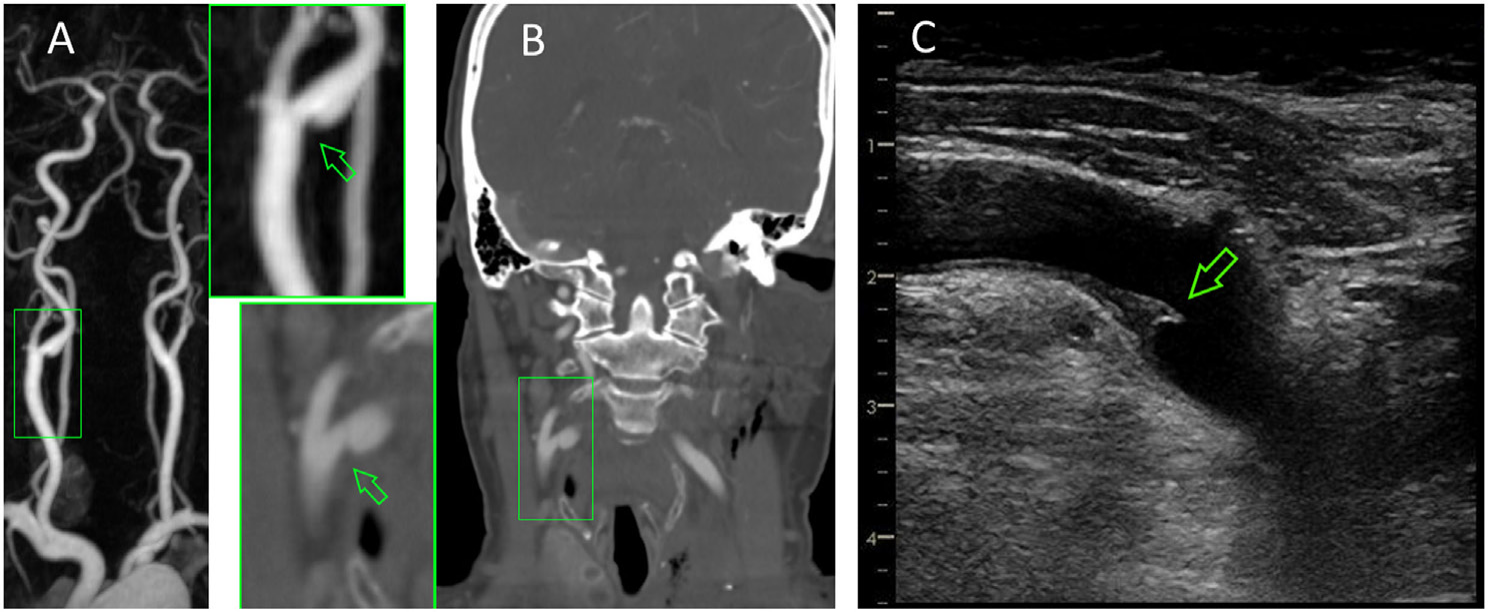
Irregular-shaped plaque formation at the origin of the ipsilateral internal carotid artery (arrows) as seen in contrast-enhanced MRA (A), computed tomography angiography (B), and neurosonography (C).
